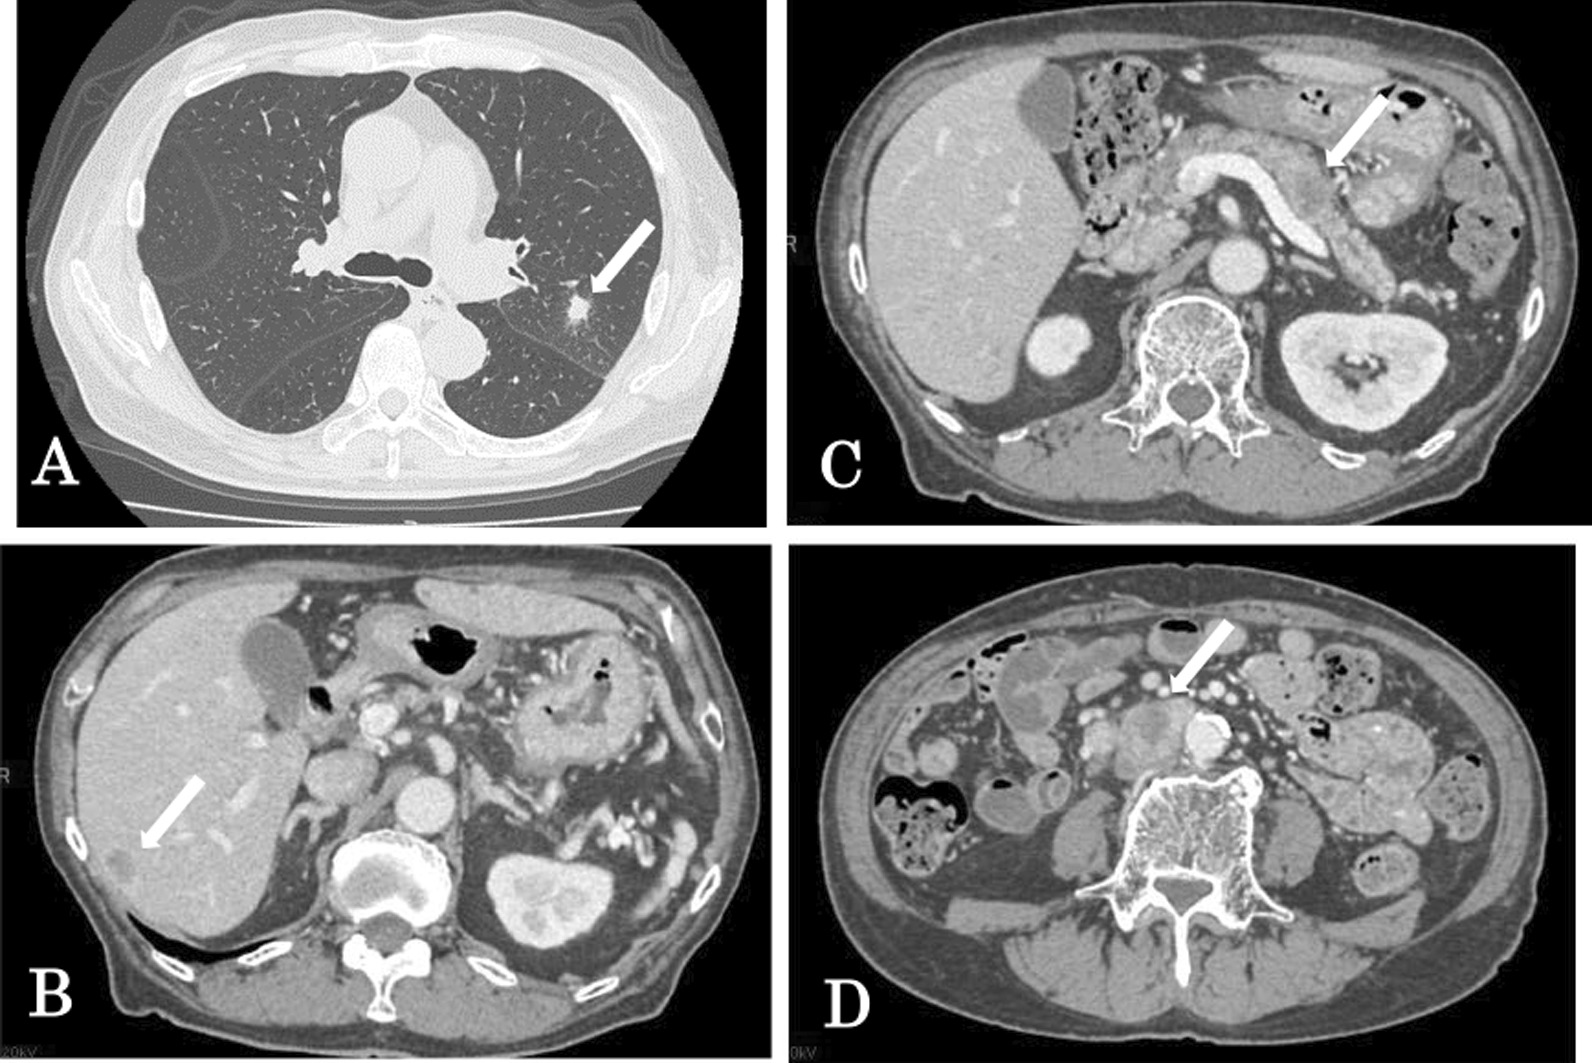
Contrast-enhanced CT after four courses of etoposide and cisplatin chemotherapy showed new metastatic lesions in the lung (A, 14 × 10 mm^2^, white arrow), liver (B, 16 × 12 mm^2^, white arrow), and pancreas (C, 18 × 15 mm^2^, white arrow), and increased para-aortic lymphadenopathy was also observed (D, 31 × 26 mm^2^, white arrow)
